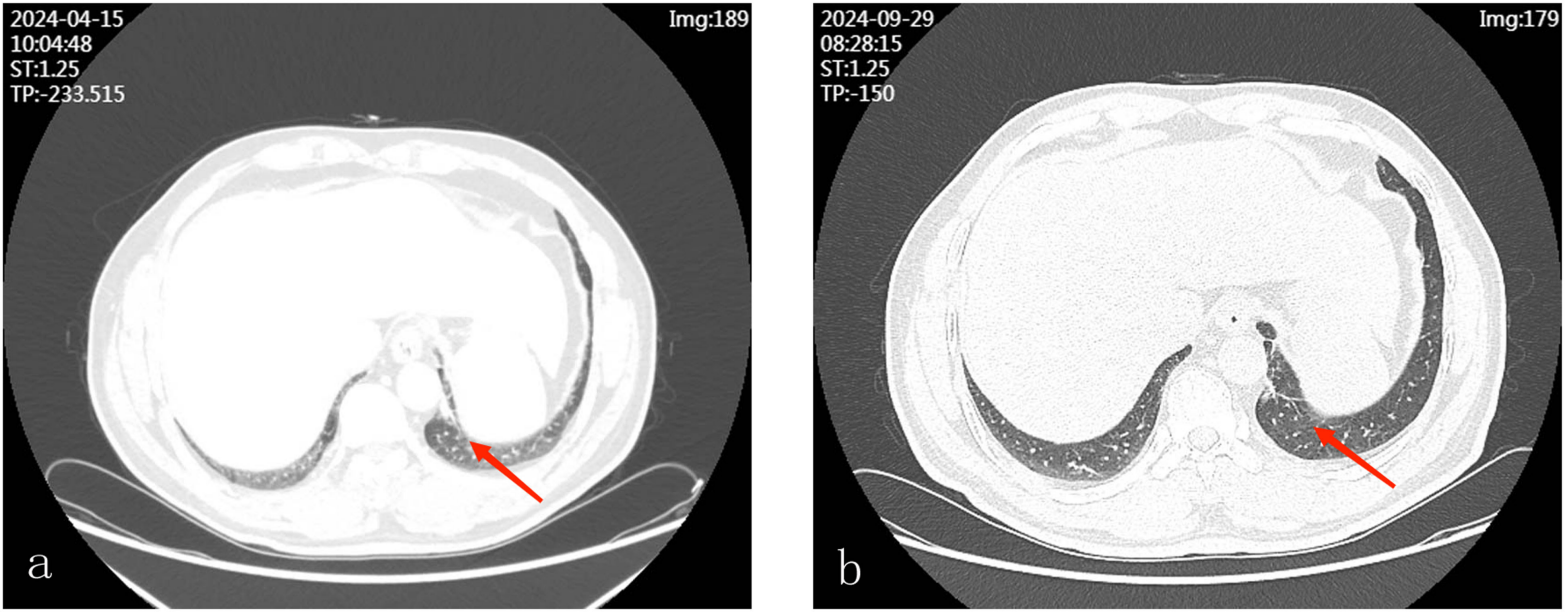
(a) Follow-up chest CT two months after targeted therapy shows a reduction in the size of the solid nodule in the lower lobe of the left lung, now approximately 0.7 × 0.7 cm. (b) Follow-up chest CT seven months after treatment shows near-complete resolution of the left lung nodule.
